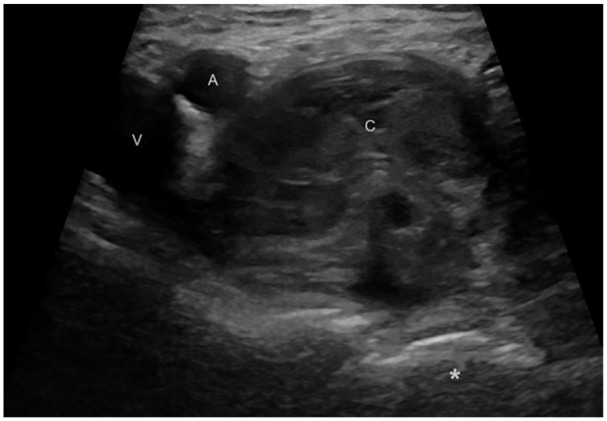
Ultrasound image, cross-sectional view at the level of the inguinal ligament. The femoral artery (a) and common femoral vein (v) are identified, along with a cystic mass (c) adjacent to these structures. The femoral head is marked with an asterisk (*).
